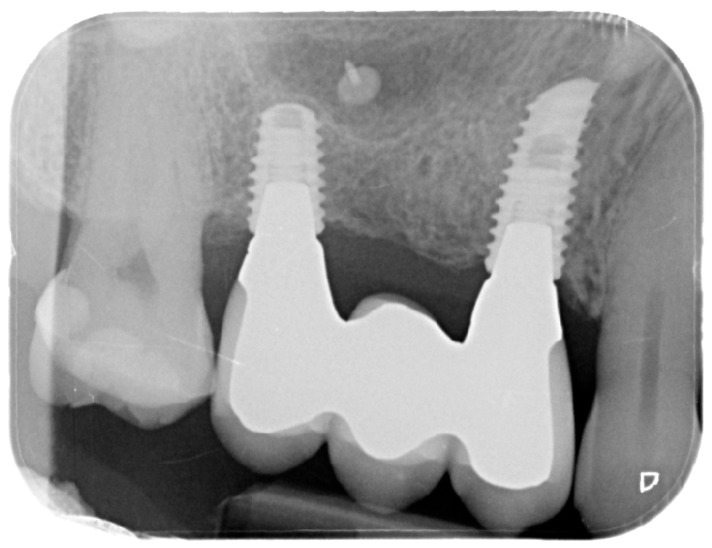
Intraoral X-ray at delivery of the final prosthesis.
